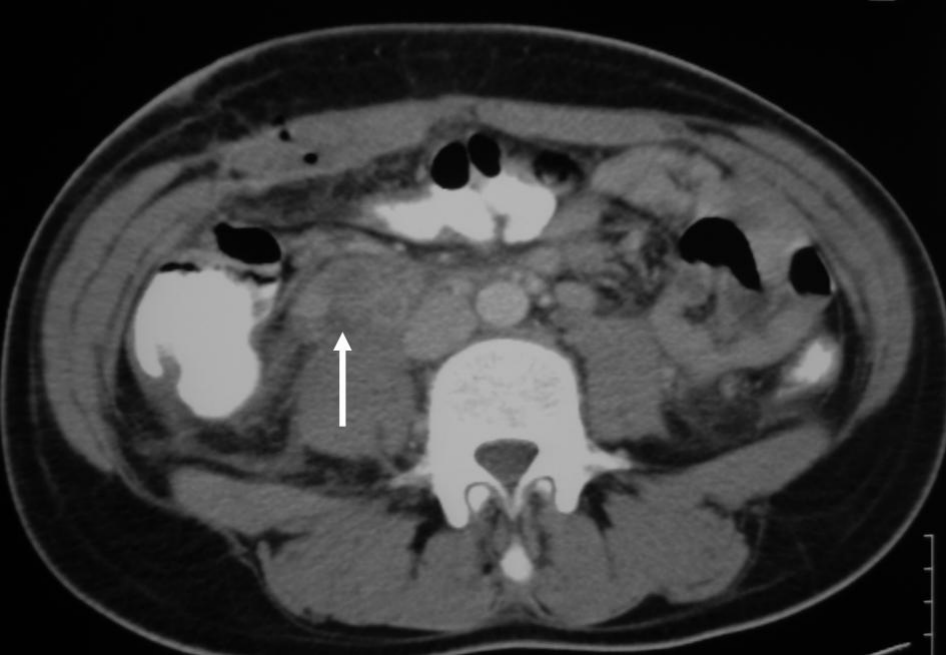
Abdominal CT scan-arrow showing thrombosed right ovarian vein.
